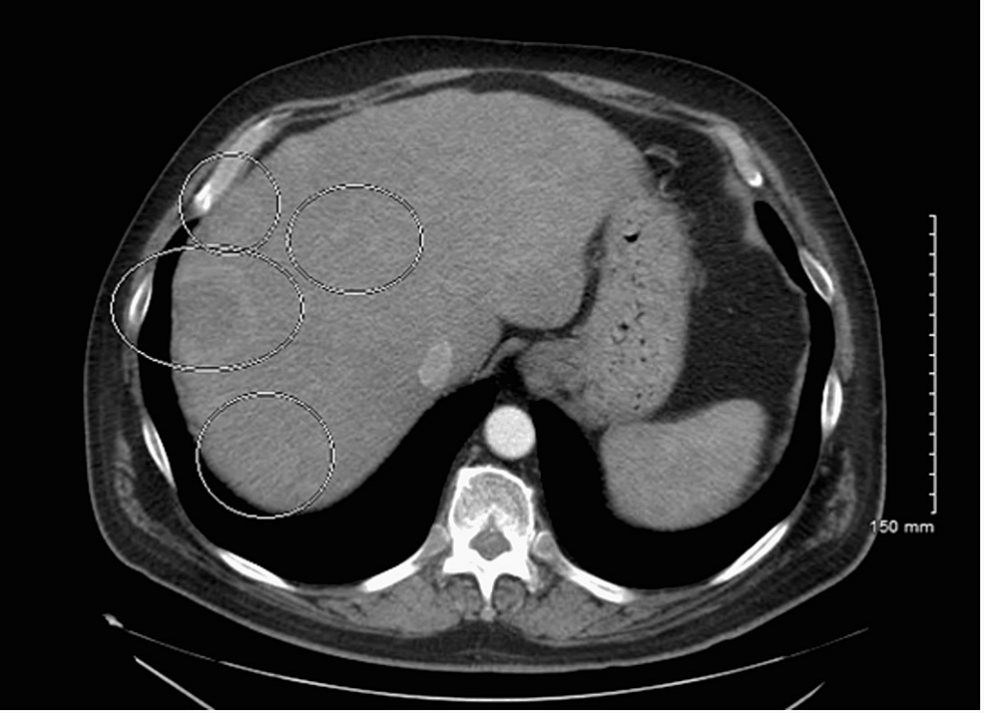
CT of the abdomen and pelvis in May 2020. CT of the abdomen and pelvis in May 2020 demonstrating metastatic liver lesions. CT: computed tomography
